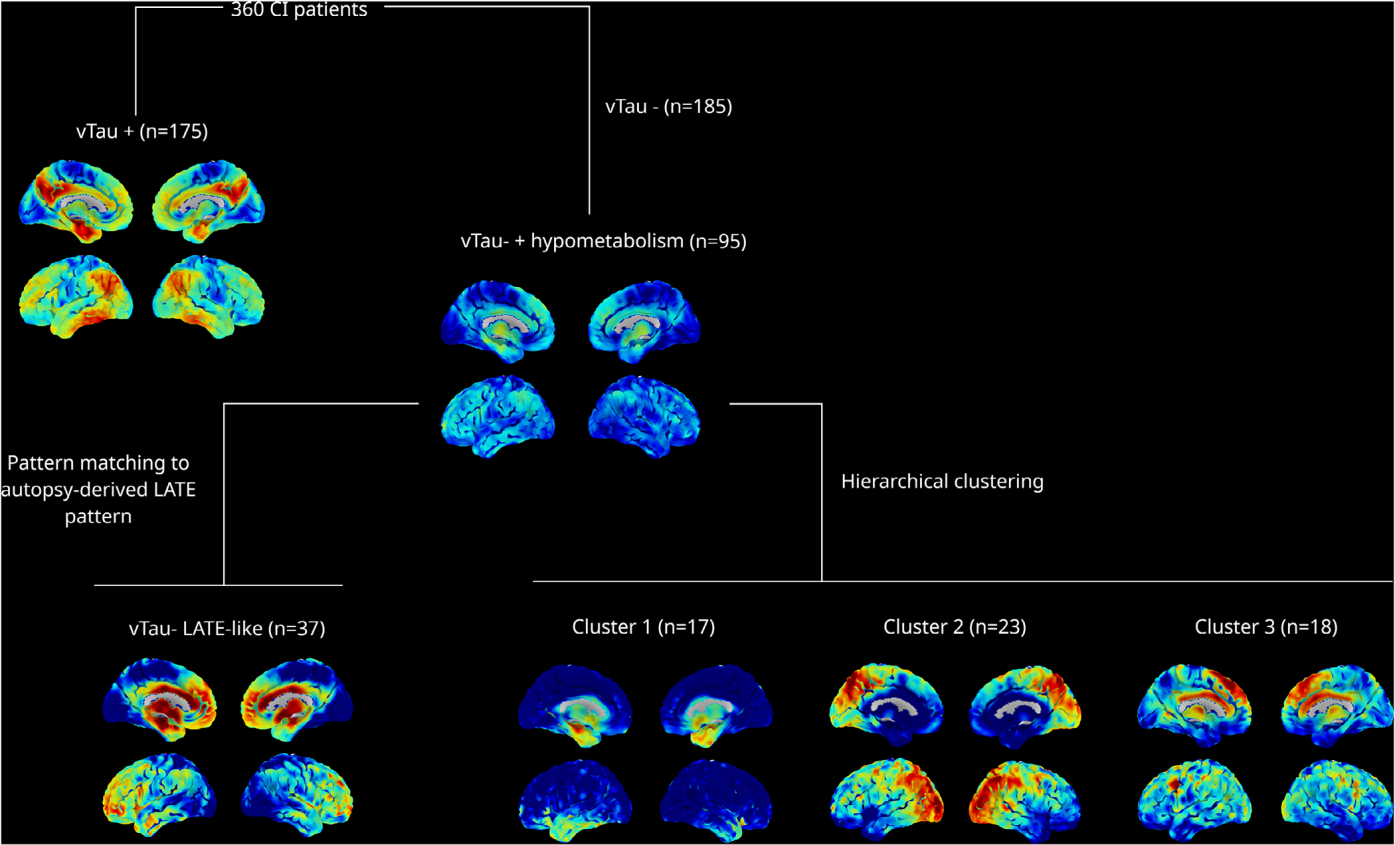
# FDG‐PET patterns of LATE & co: disentangling the pathological heterogeneity of Tau‐negative amnestic syndromes
